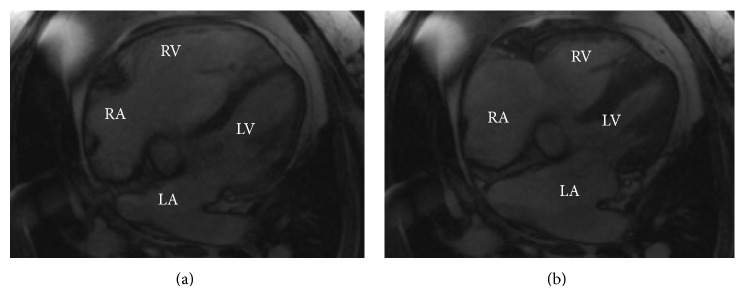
Long axis cardiac magnetic resonance imaging prior to surgical correction. Panel (a) demonstrates severe right ventricular enlargement during end-diastole of the cardiac cycle. Panel (b) demonstrates severe right atrial enlargement during end-systole of the cardiac cycle. RA: right atrium; RV: right ventricle; LA: left atrium; LV: left ventricle.
